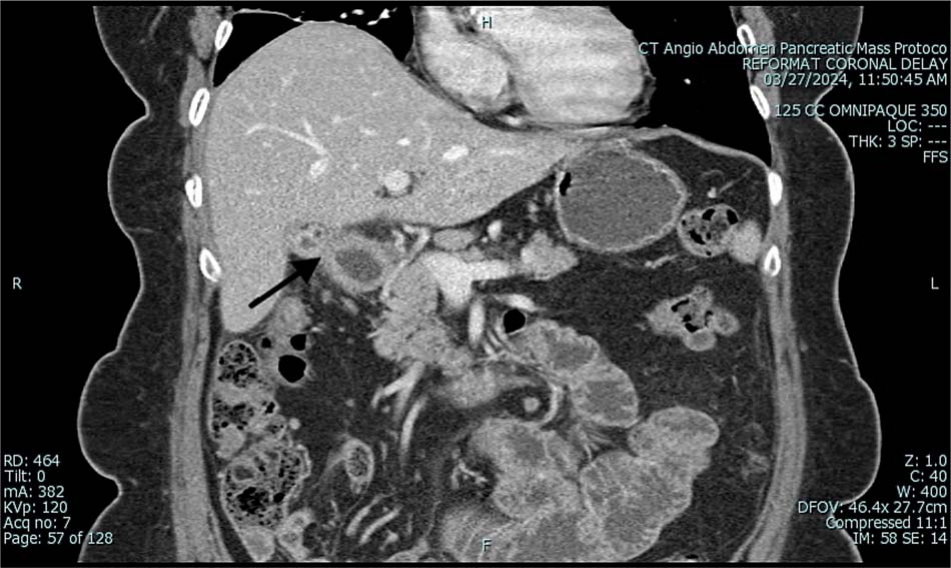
Postoperative CT of abdomen in coronal view, demonstrating suspected cholecystoduodenal fistula.
